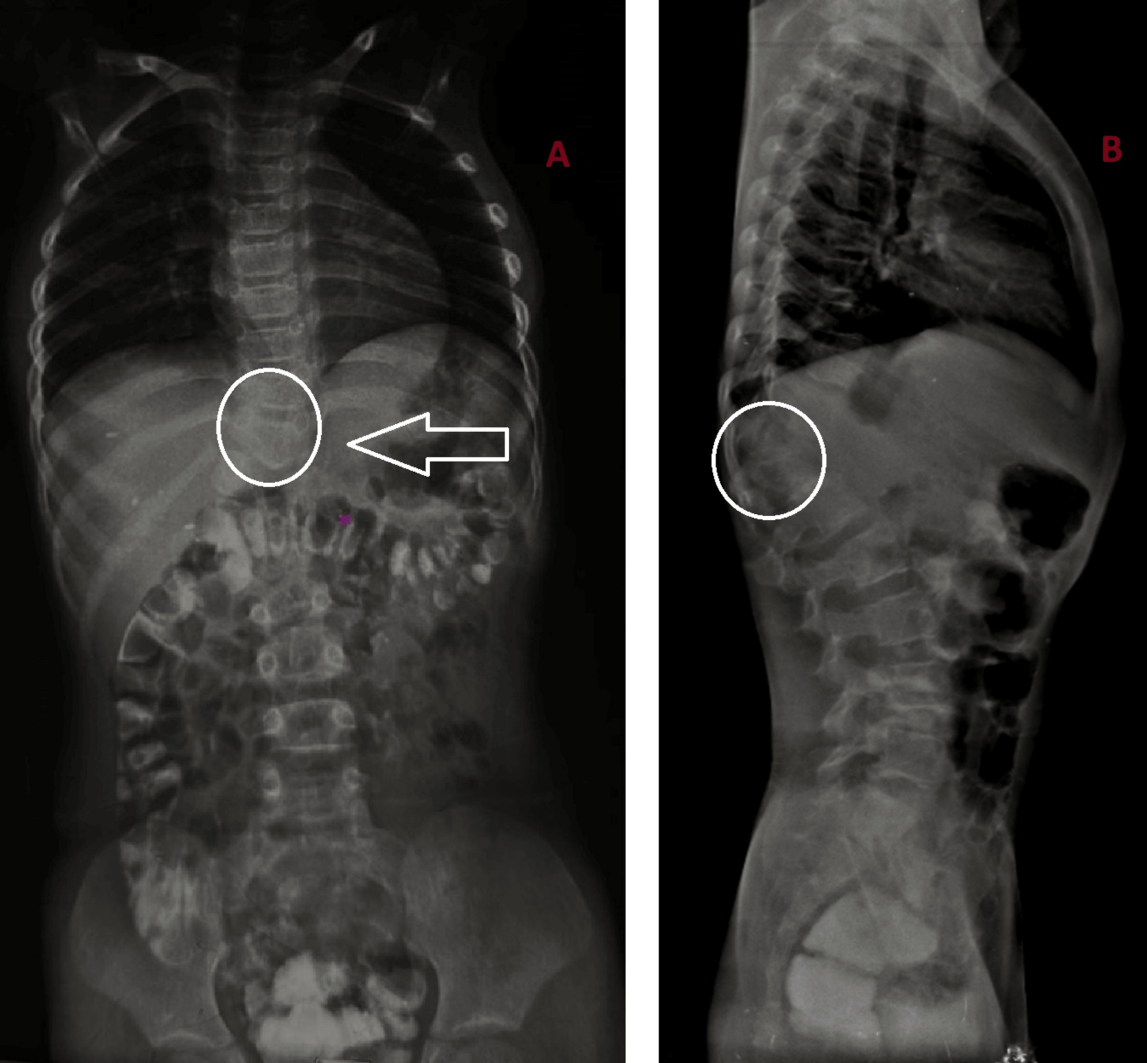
Frontal (A) and lateral (B) X-ray abdomen showing dorsal vertebral anomalies and dextroscoliosis (circle) with absence of left 12th rib (arrow)
